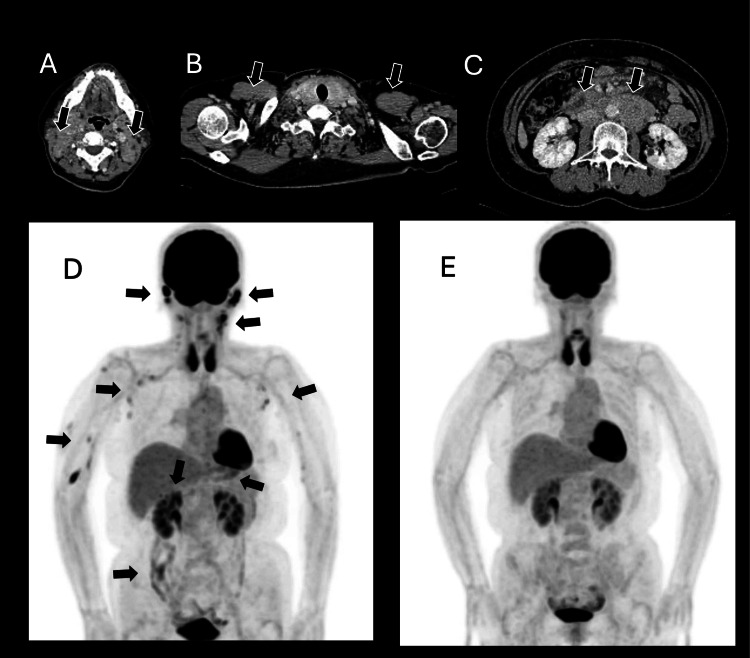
The follicular lymphoma computed tomography (CT) and fluorodeoxyglucose F18 positron emission tomography (FDG-PET). At the onset, the patient had lymph node swellings in the bilateral cervix and larynx ((A) arrows), axilla ((B) arrows), and abdomen ((C) arrows). Nine and a half years after the onset, the lymphoma relapsed. The lymph nodes and organization in the face, neck, parotid glands, and axilla were swollen in FDG-PET ((D) arrows). The FDG-PET confirmed the remission of lymphoma after the sixth injection of epcoritamab (E).
